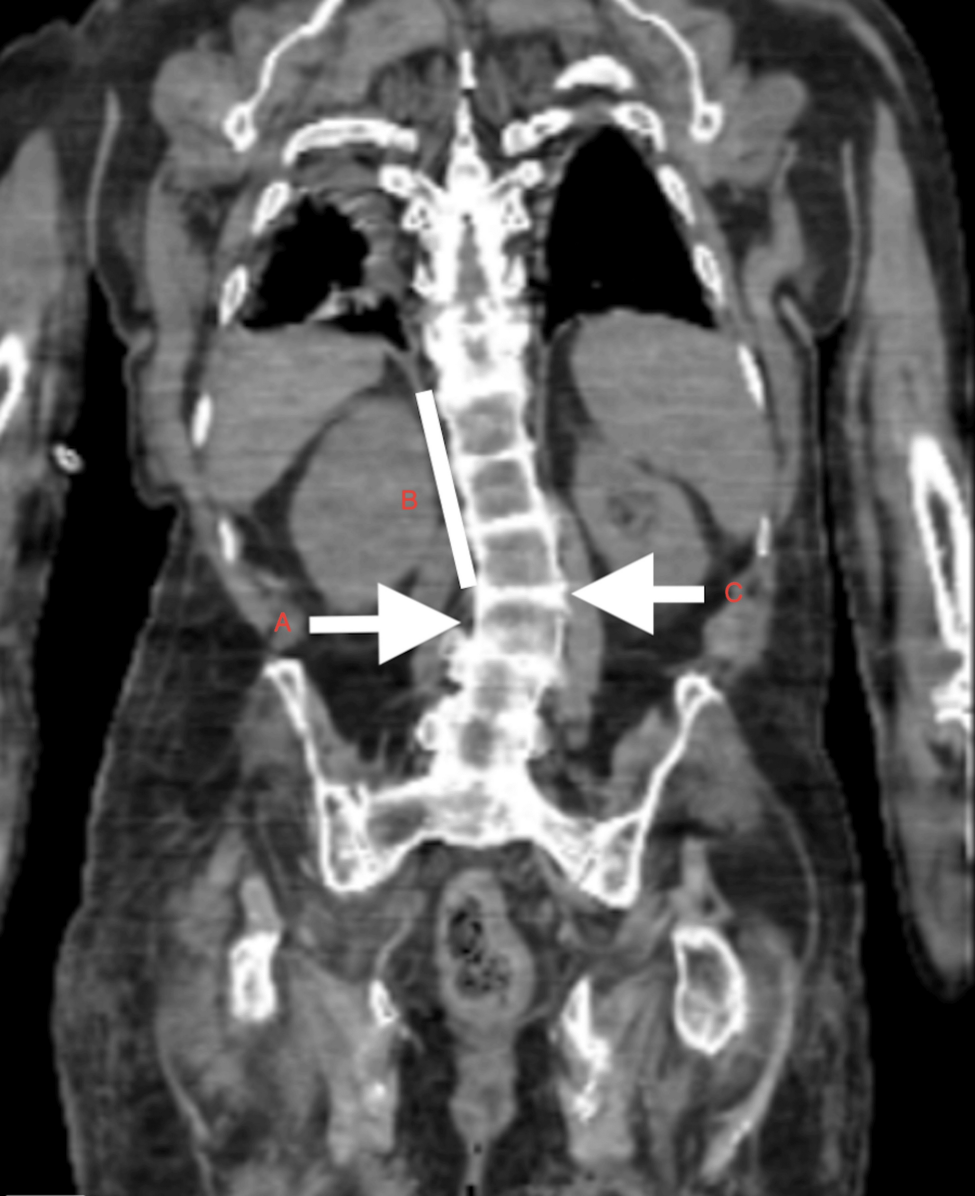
CT of dorso-lumbar spine showing A) dorso-lumbar scoliosis, B) ankylosis, diffuse osteopenia, disc space narrowing and C) multiple intervertebral disc space calcification and osteophytes
